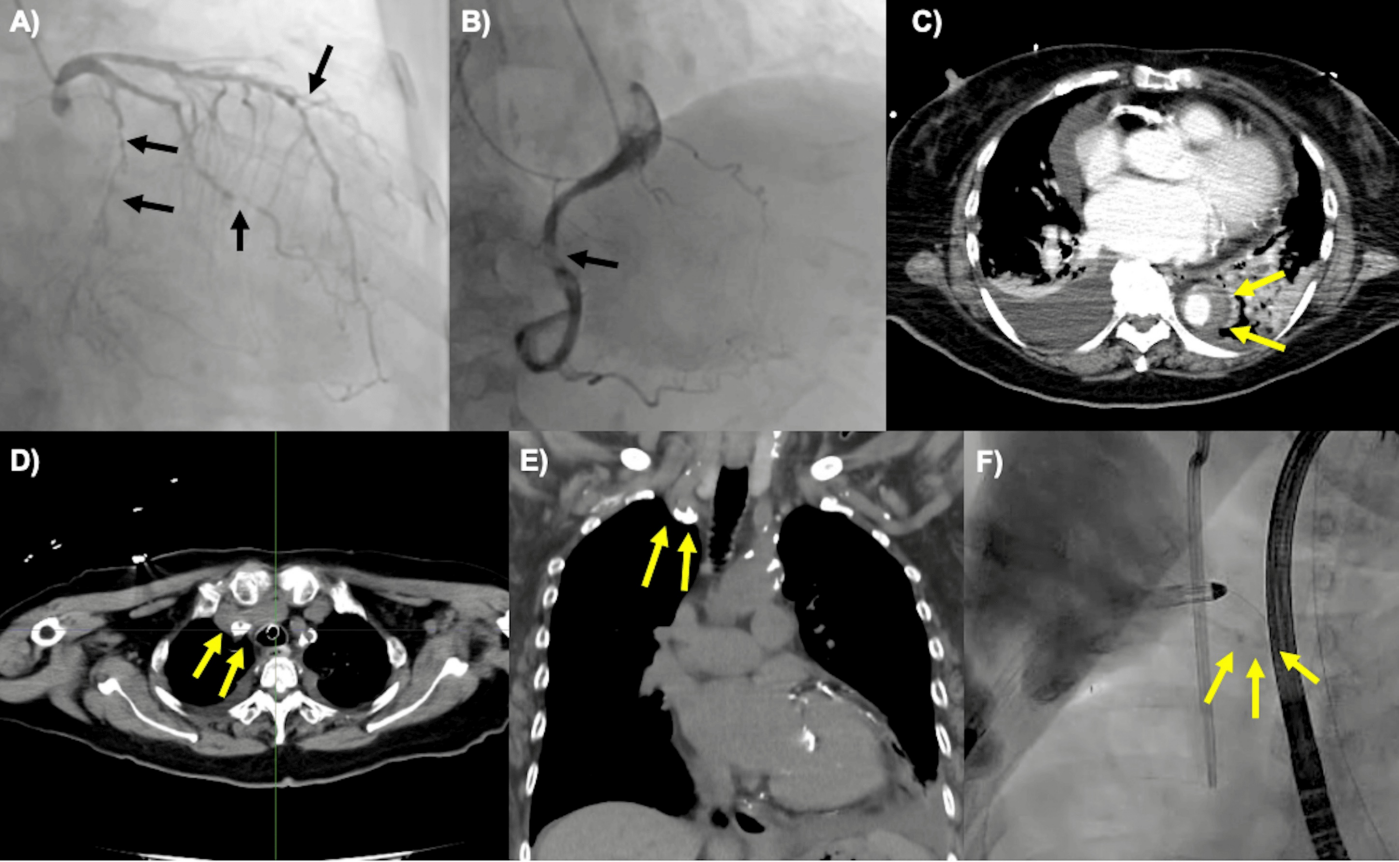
Preoperative CAG, CT, and intraoperative findings during IMPELLA 5.5 insertion (A, B) CAG shows significant stenosis. The black arrows indicate 75% stenosis of the left anterior descending artery, 75-90% stenosis of the left circumflex coronary artery, and 90% stenosis of the right coronary artery. (C) CT reveals a thrombosed type B aortic dissection (yellow arrows). (D, E, F) CT and intraoperative angiography demonstrate severe calcification of the right subclavian artery, as indicated by the yellow arrows. The calcification extended into the vascular lumen (E). CAG: coronary angiography IMPELLA 5.5; Abiomed Inc., Danvers, Massachusetts, United States
